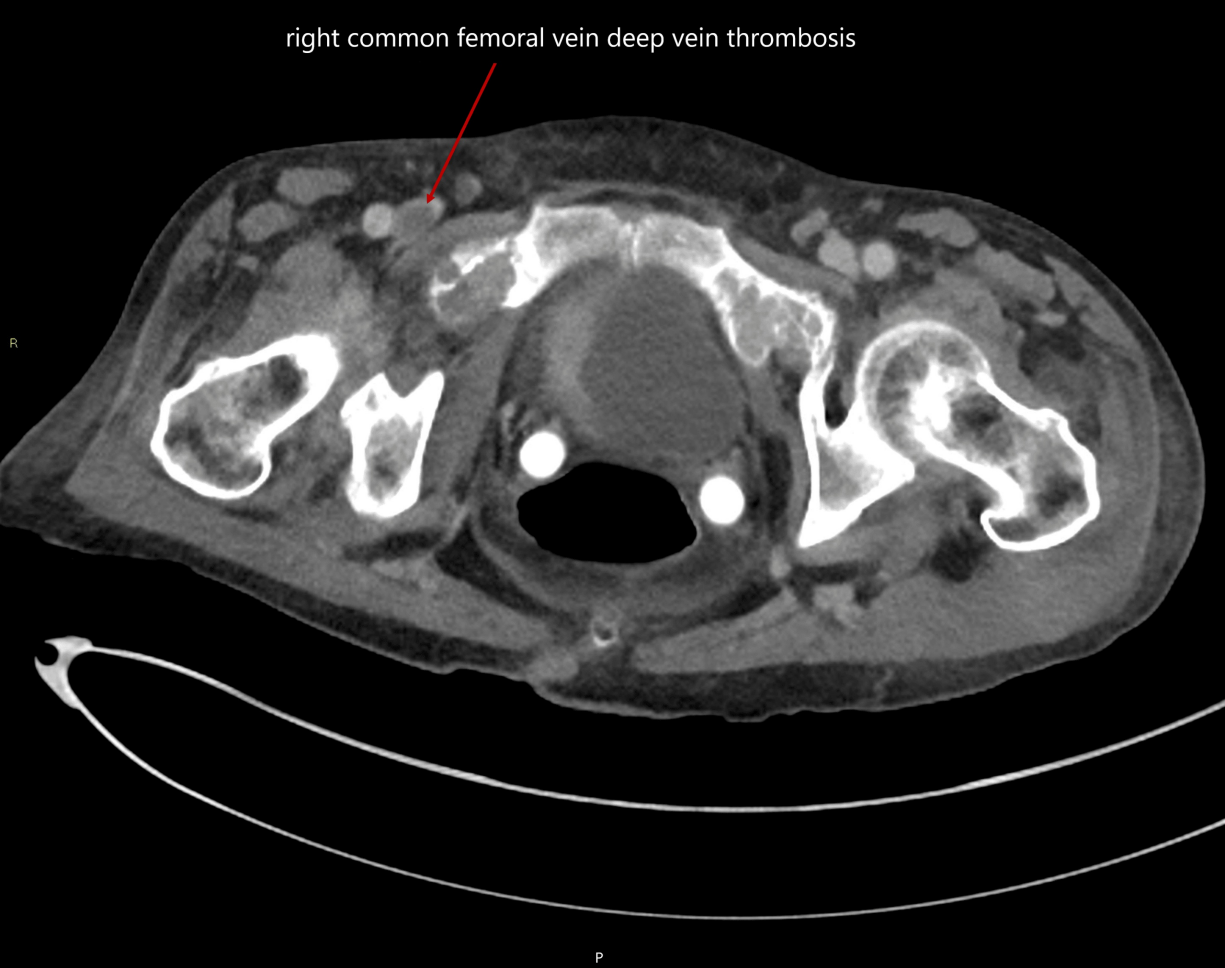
CT of the chest, abdomen, and pelvis with contrast, axial section, showing a right common femoral vein deep vein thrombosis CT: computed tomography
